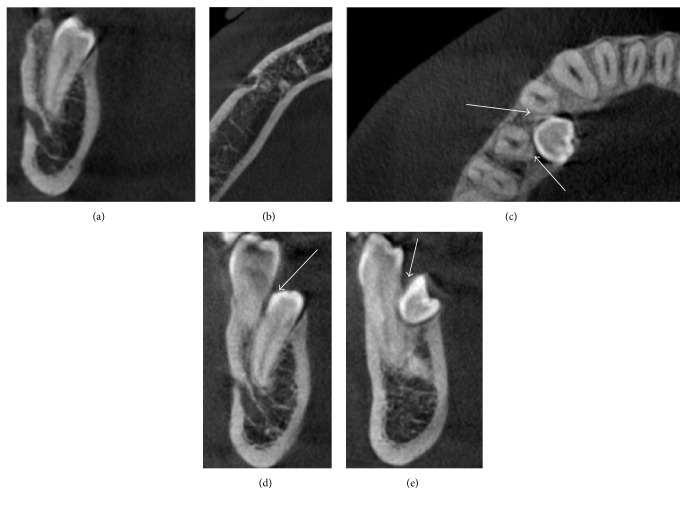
Case 3 CBCT: no continuity between the ST's root and the alveolar nerve (a, b) and root resorption of contiguous teeth, indicated by the white arrows (c–e).
